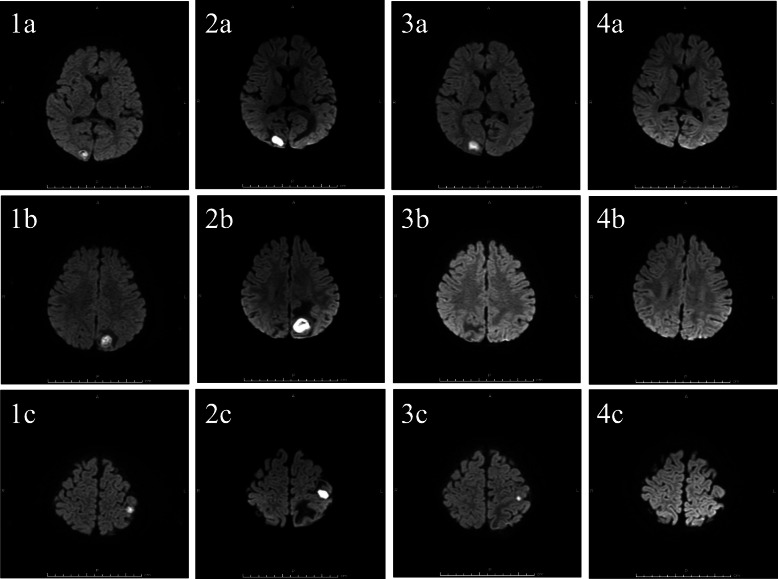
Clinical course of the lesions as seen on diffusion-weighted magnetic resonance images on the day of admission (1a–1c), at 8 days after admission (2a–2c), at 13 days after surgical aspiration (3a–3c), and at 4.5 months after onset (4a–4c). Very hyperintense lesions were revealed in the right occipital lobe (1a–4a), left occipital lobe (1b–4b), and left parietal lobe (1c–4c) on the day of admission (1a–1c). These lesions all enlarged before surgical aspiration (2a–2c). The left occipital lesion disappeared immediately (3b), and the other lesions reduced after surgical aspiration (3a, c) and eventually disappeared (4a, c)
